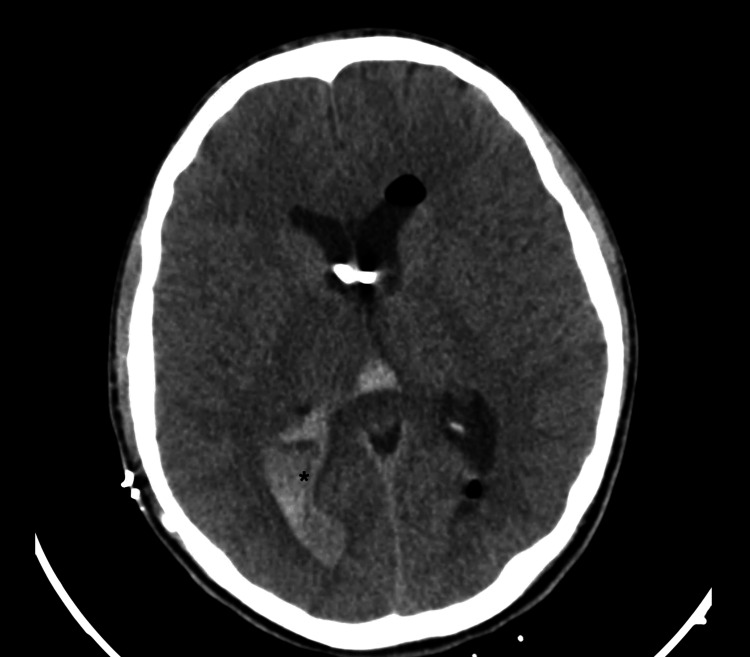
Post-surgical CT scan showing significant intraventricular hemorrhage (*).
